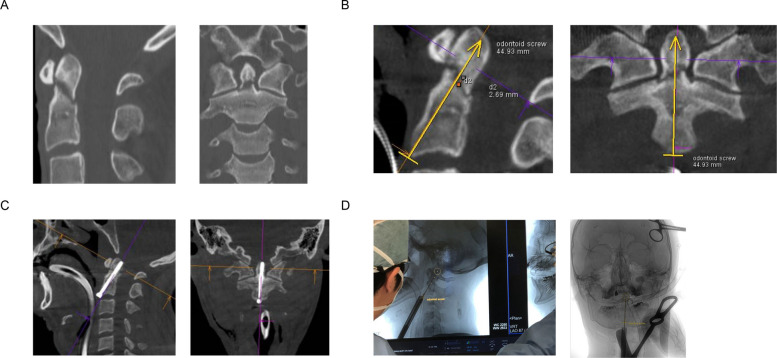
Overlay technology process (case 1). A Pre-operation C-arm cone beam computed tomography (CT) images. B Planning and determination of needle guidance. C Post-operation C-arm cone beam CT images. D Overlay image during operation
